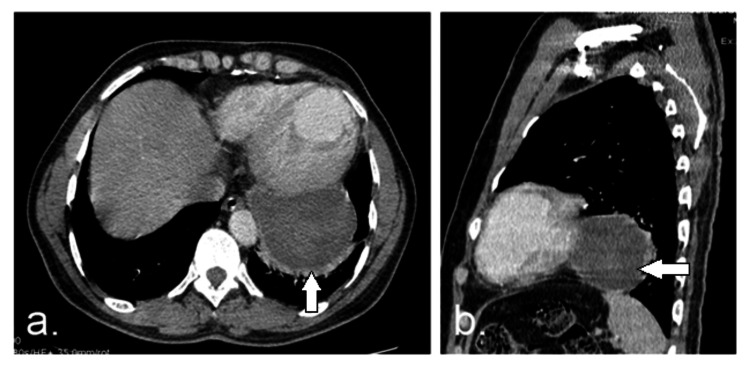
(a) axial and (b) sagittal contrast-enhanced CT (mediastinal window) images show non-enhanced fluid density lesions located in the left posterior cardiopheric angle in close proximity to the pericardium (white arrow) with associated compressive atelectasis of adjacent pulmonary segments
